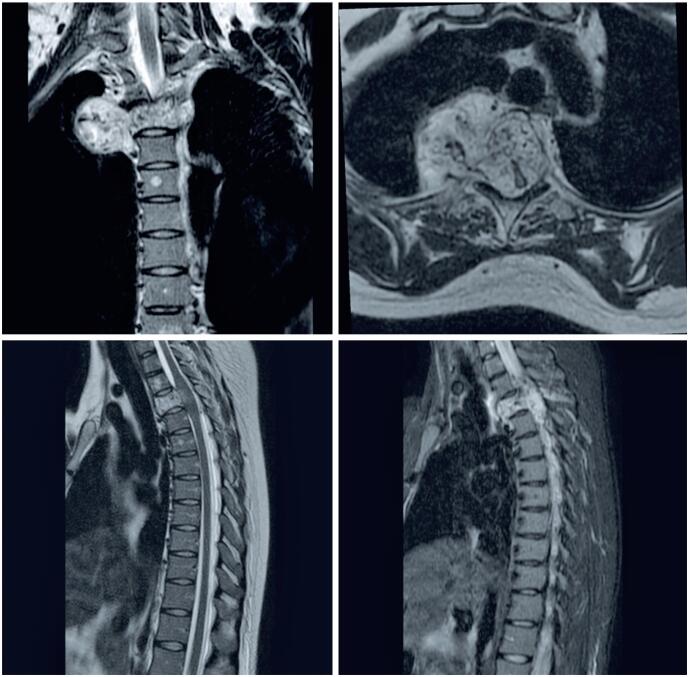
Magnetic resonance imaging of the thoracic spine. Solid neoplastic mass at the T3 level, with diffuse vertebral body infiltration, vertebral canal invasion, and extraosseous paravertebral and foraminal extension, resulting in spinal cord compression
